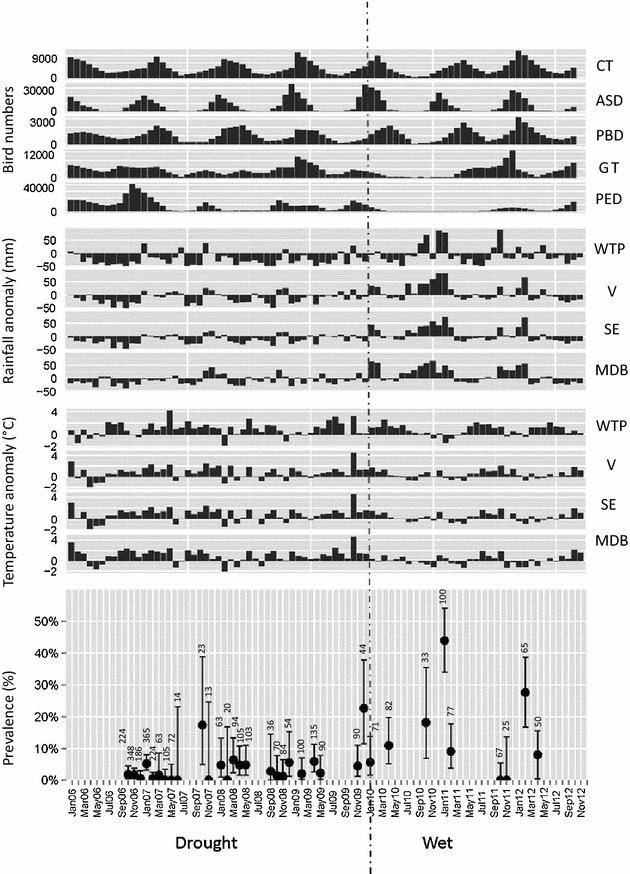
Bird numbers of five waterfowl species, rainfall anomaly and temperature anomaly for four geographic regions, and AIV prevalence with 95% confidence intervals between 2006 and 2012. The data is subdivided into a drought (2006–2009) and wet (2010–2012) period. The bird species for which data are presented are Chestnut Teal (CT), Australian Shelduck (ASD), Pacific Black Duck (PBD), Grey Teal (GT) and Pink-eared Duck (PED). The four geographic regions are Western Treatment Plant (WTP), Victoria (VIC), South-eastern Australia (SE) and Murray–Darling Basin (MDB).
